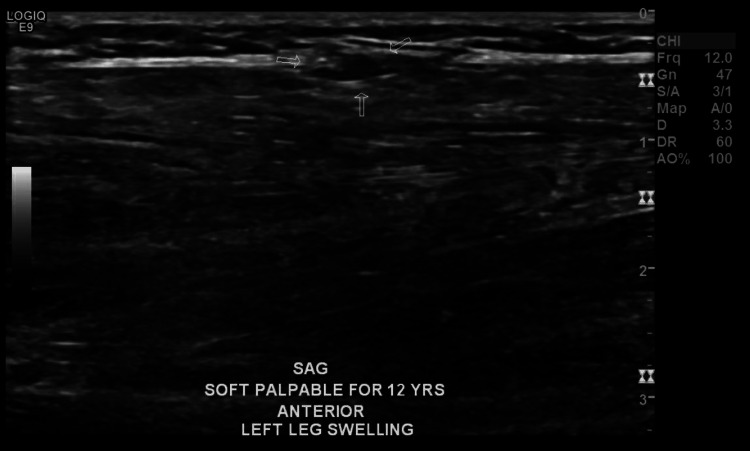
Ultrasound revealing the herniated muscle (arrows)
